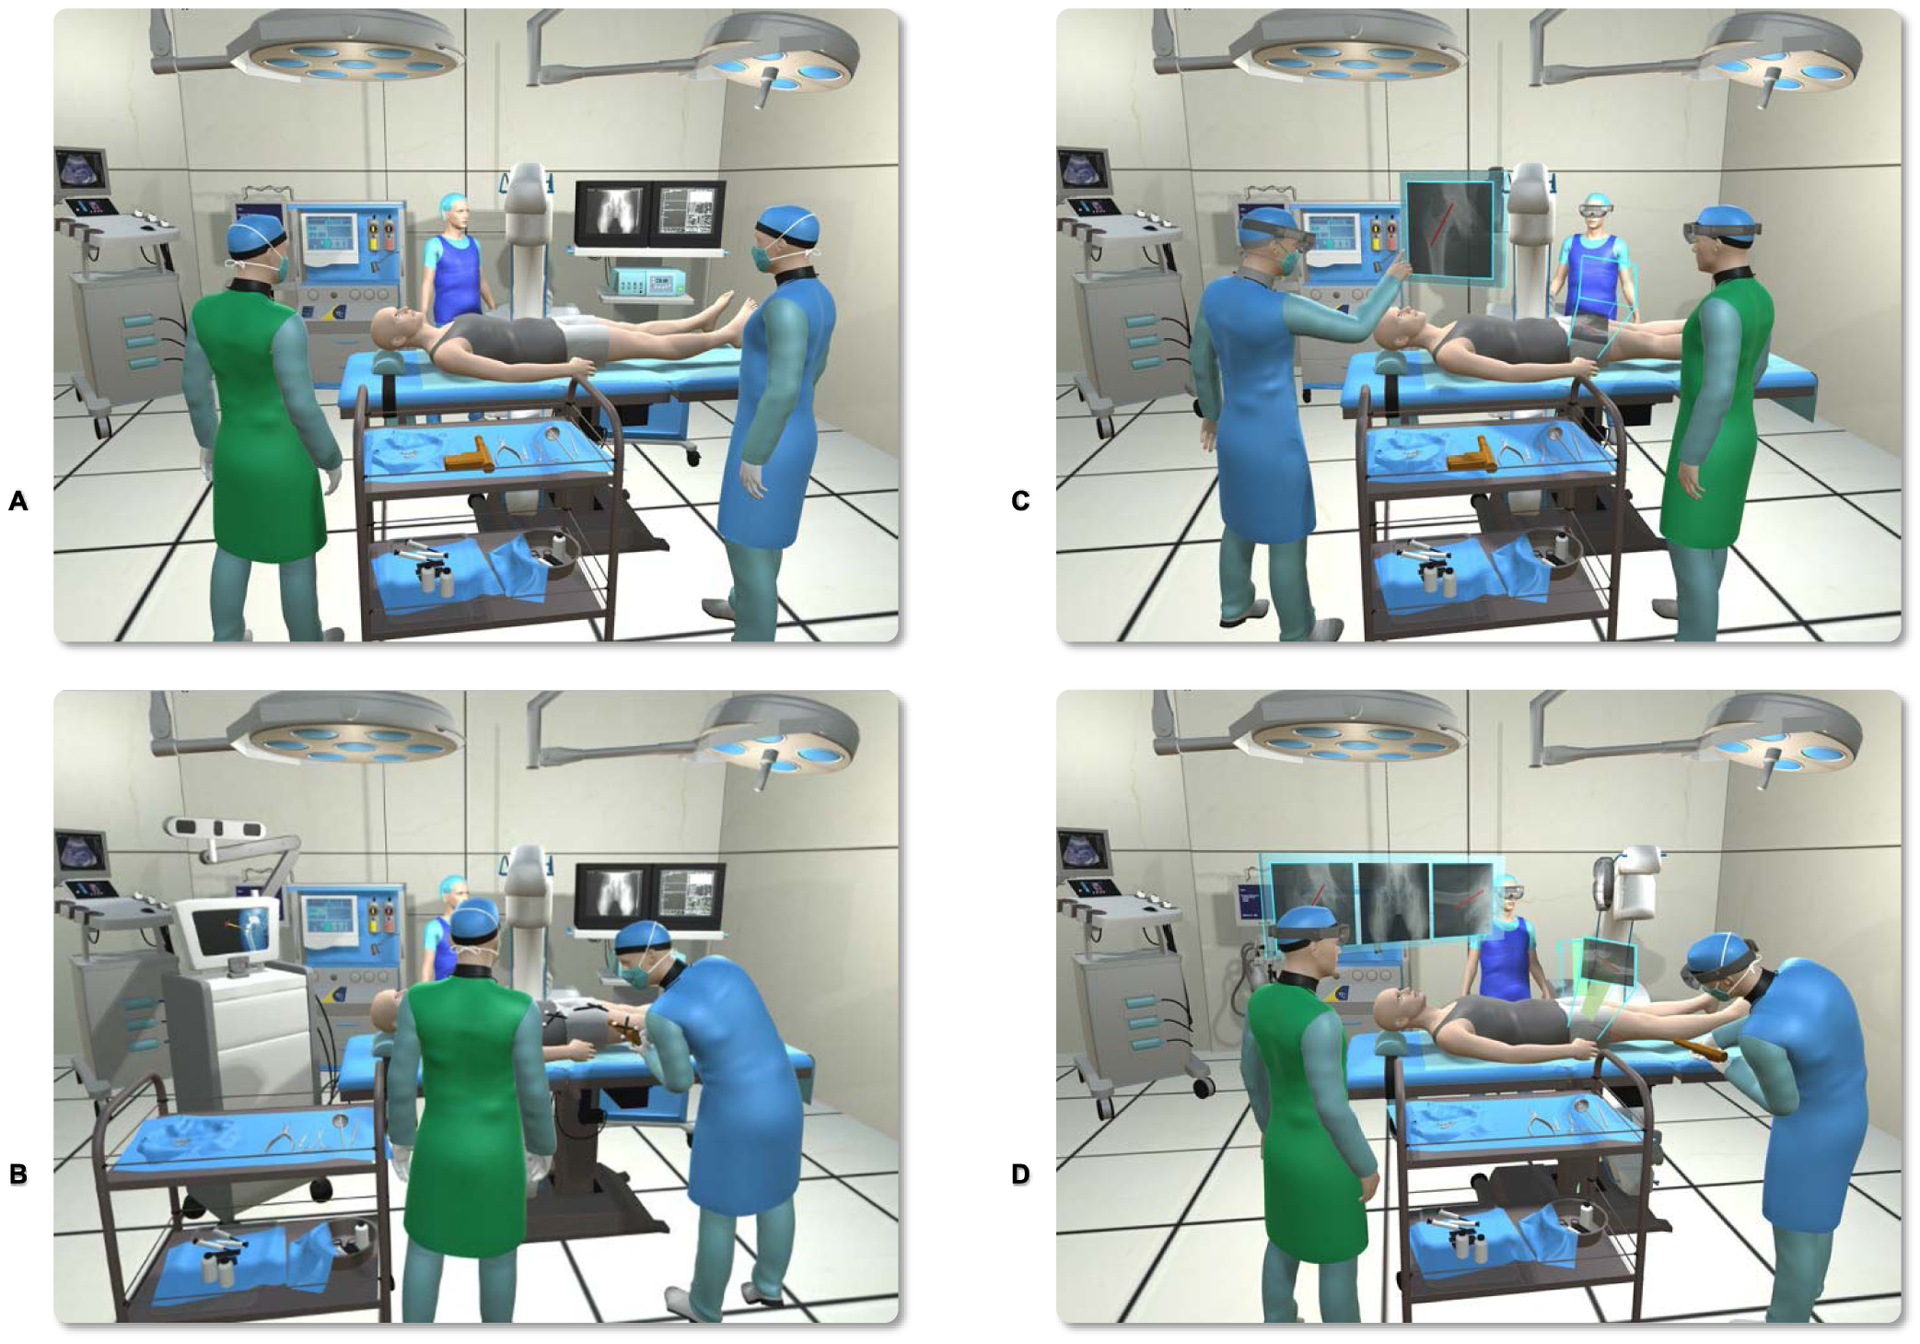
The standard operative procedure in percutaneous orthopedic interventions makes extensive use of interventional imaging (A). Classic navigation-based solutions use sophisticated tracking hardware and external markers to provide geometric registration between the content in the image and the patient (B). On the other hand, in the AR-enhanced OR that we suggest (C), the surgeon and crew interactively use the data and pass the information around without explicit navigation. Based on the concepts introduced in Sec. II-D, the planning on X-ray images is directly visualized on the patient. The surgeon takes action based on the information from planning, as well as the X-ray images that are positioned within their respective frustums, both of which are seen through the HMD (D).
